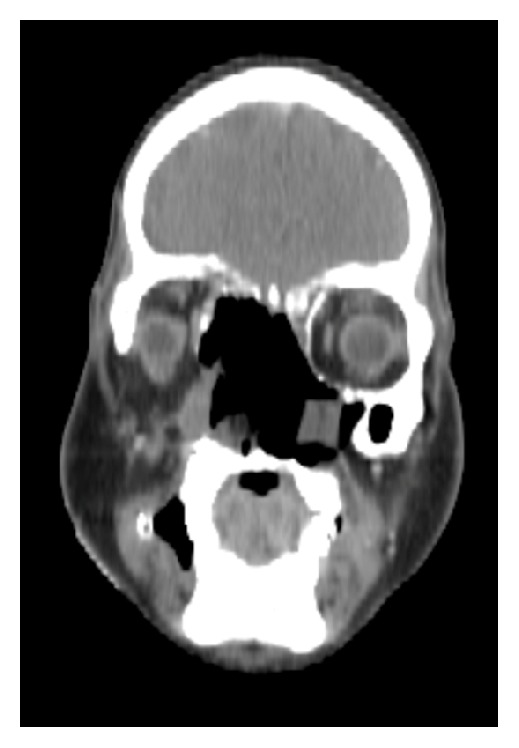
Coronal cut of contrast enhanced CT paranasal sinus showing postoperative changes and absence of any recurrence.
